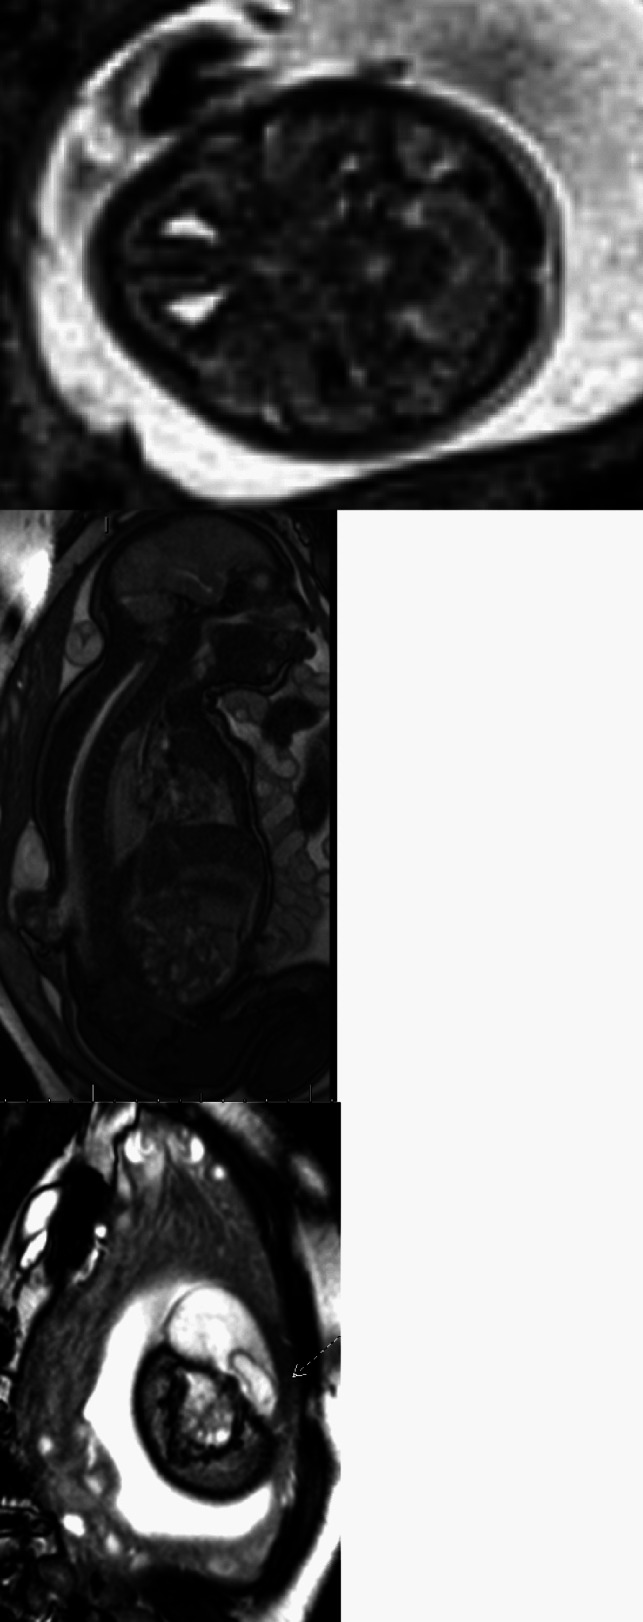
Prenatal ultrasonographic images showing lumbosacral neural tube defect
